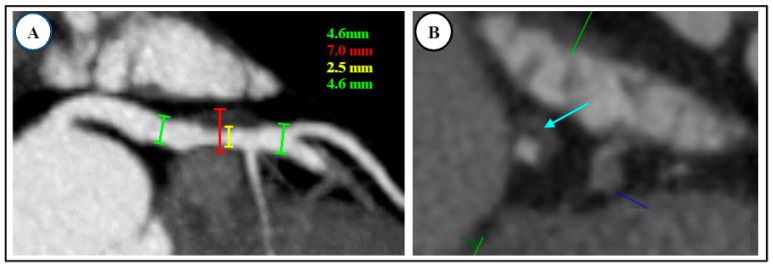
High-risk plaque features on coronary CT angiography (CAD RADS = 3 + HRP). HRP = High-risk plaque. (A) Positive remodeling seen in proximal left anterior descending artery (red bracket), with moderate (50–60%) underlying stenosis (2.5 mm luminal diameter shown with the yellow line). Outer caliber of artery is >1.5 times the inner lumen; 7.0 mm total vessel diameter is shown with the red line, and the green lines with 4.6 mm measurements demonstrate the luminal diameter pre- and post-stenosis. (B) Low-attenuation lipid core (<30 Hounsfield units, light blue arrow) with speckled calcium.
